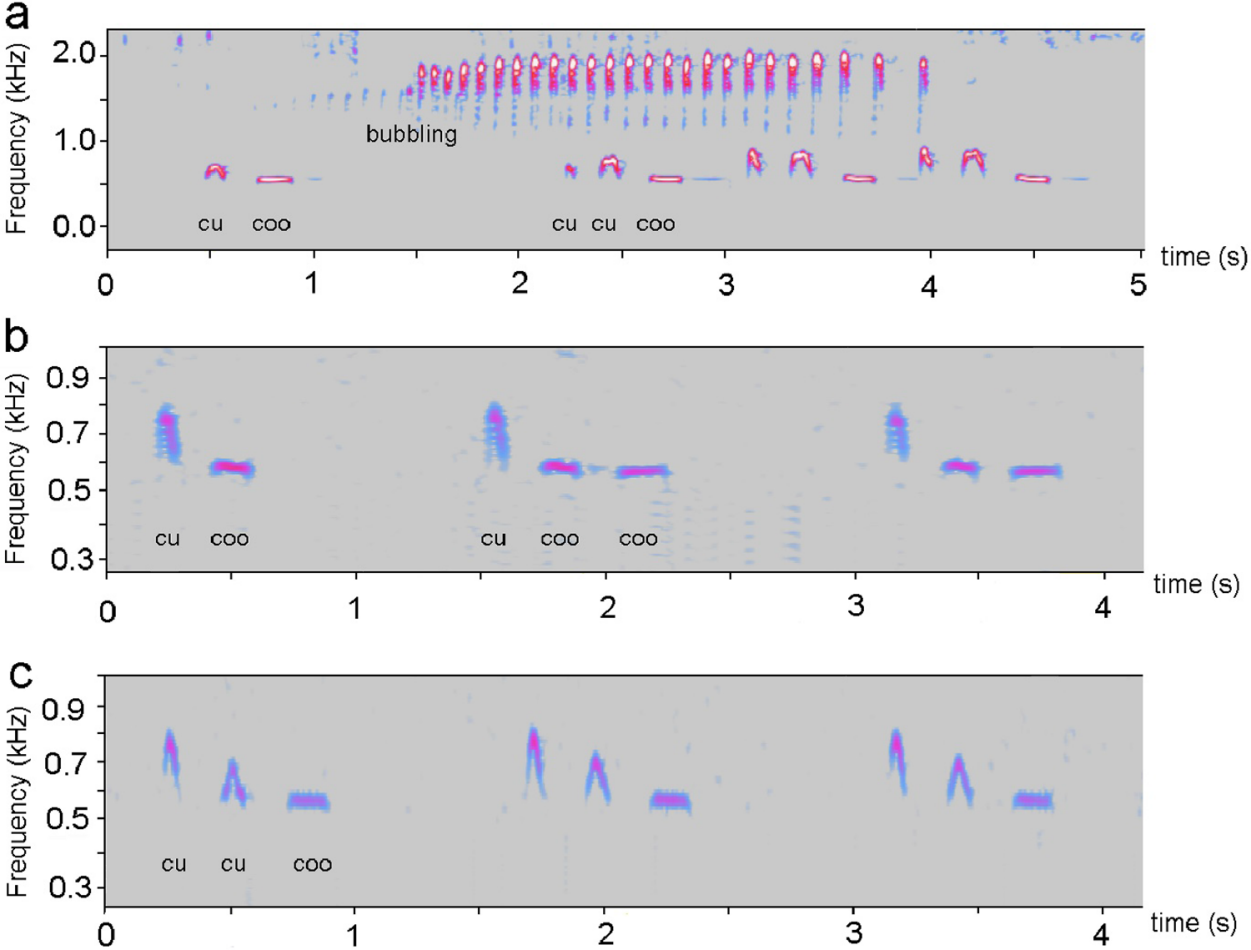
Examples of male (and female) common cuckoo calls. (a) The natural forms of 2-note (“cu-coo”) and 3-note (“cu-cu-coo”) calls of males, together with a female-specific bubbling call also indicated on the sonogram. (b) A “cu-coo” call and the first two calls in a series of “cu-coo” calls with a syntactic error, where the second note is repeated (the “coo” element, i.e. ”cu-coo-coo”). (c) A normal “cu-coo” call and calls from a series of “cu-cu-coo” syntax errors. A proportion of (b,c) were uttered by the same individual cuckoo. Note that the natural 3-note call in (a) is normal (natural), but the 3-note call in (c) differs from it in several respect (see more details in the text): it specifically suffers from a syntactic error, where the first note of the normal “cu-coo” call (“cu”) is repeated. In (c) the decreasing frequencies of the first two “cu” elements are a unique case, showing that cuckoos are capable of frequency modulation (typically, the second note is about as high as that of first element).
